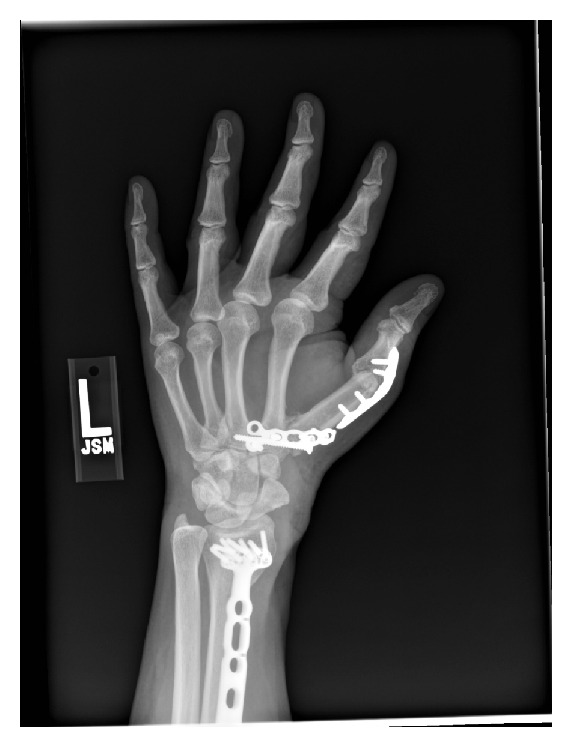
Postoperative X-ray of the left hand.
